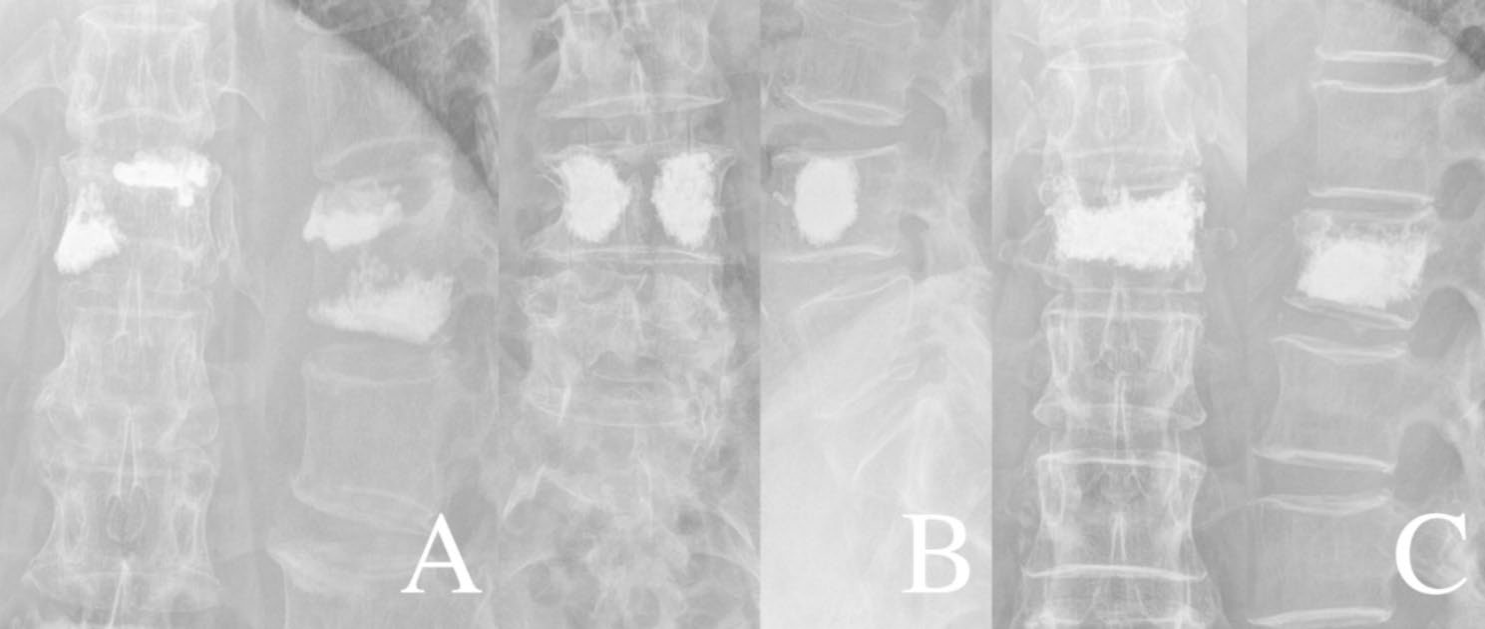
Dispersion distribution grade of bone cement. (A) The bone cement projection of the anteroposterior and lateral X-ray after surgery is ≤ ½, which is grade (1) (B) The bone cement projection of the anteroposterior was ≥ ½ and the lateral X-ray after surgery is ≤ ½, which is grade (2) (C) The bone cement projection of both the anteroposterior and lateral X-ray after surgery were ≥ ½, which is grade 3
